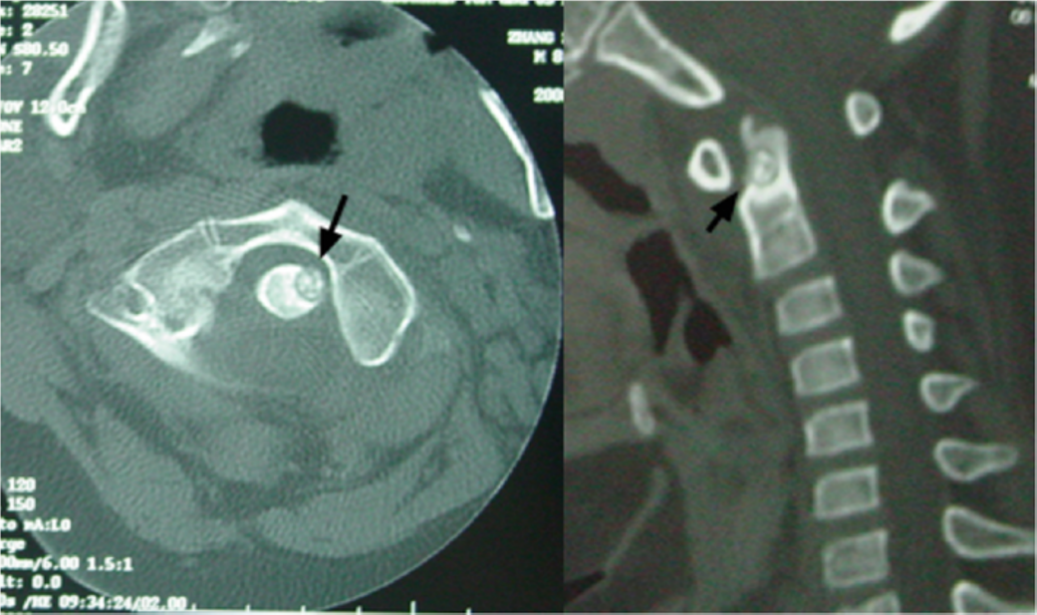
Transversal computed tomography (CT) images showing the nidus with partial ossification of matrix and sclerotic margin, and sagittal CT images of the nidus.
